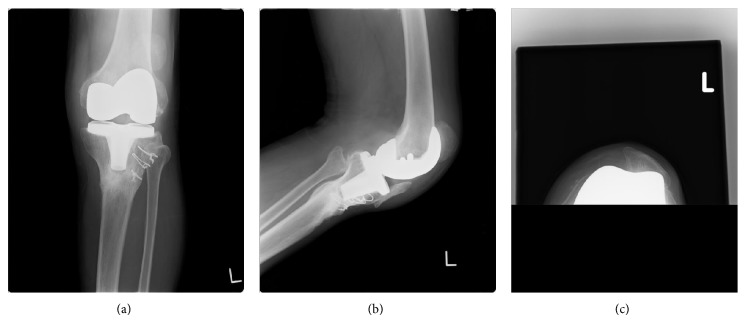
Final follow-up radiographs: anteroposterior view (a), lateral view (b), and skyline view (c) of left knee.
